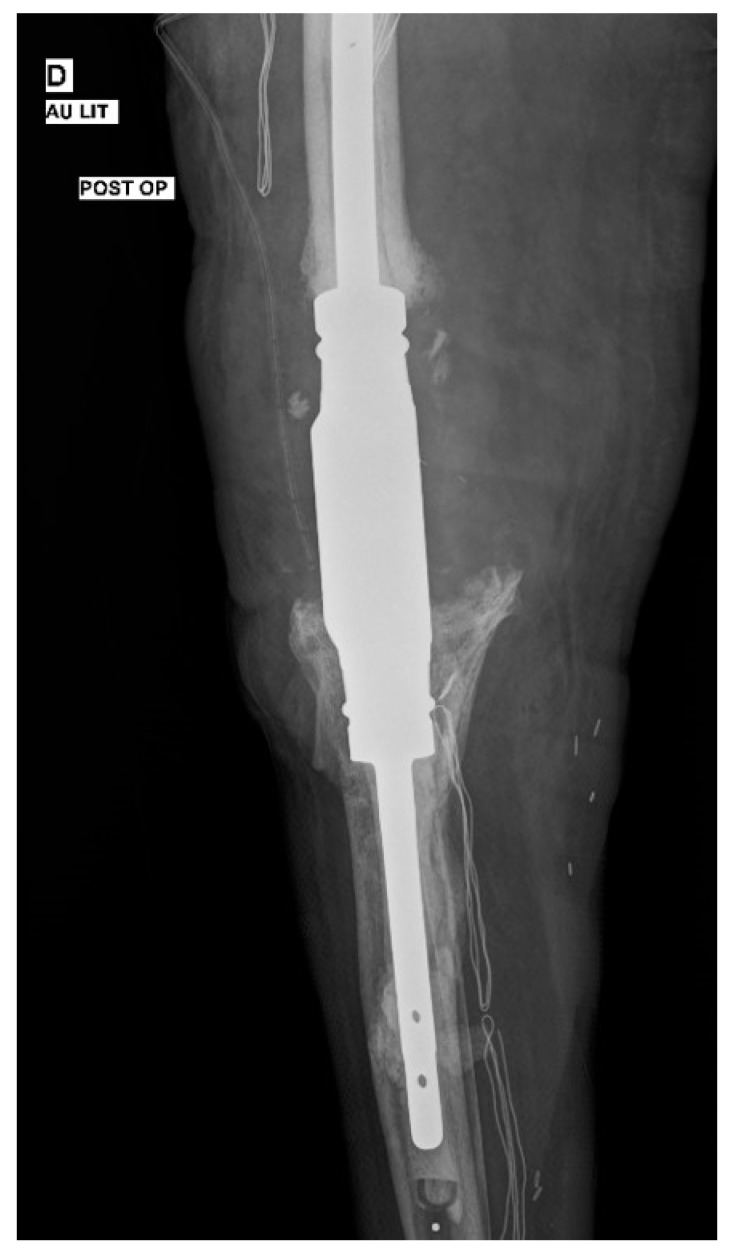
Anteroposterior post-operative radiographic view of a prosthesis-arthrodesis implant.
